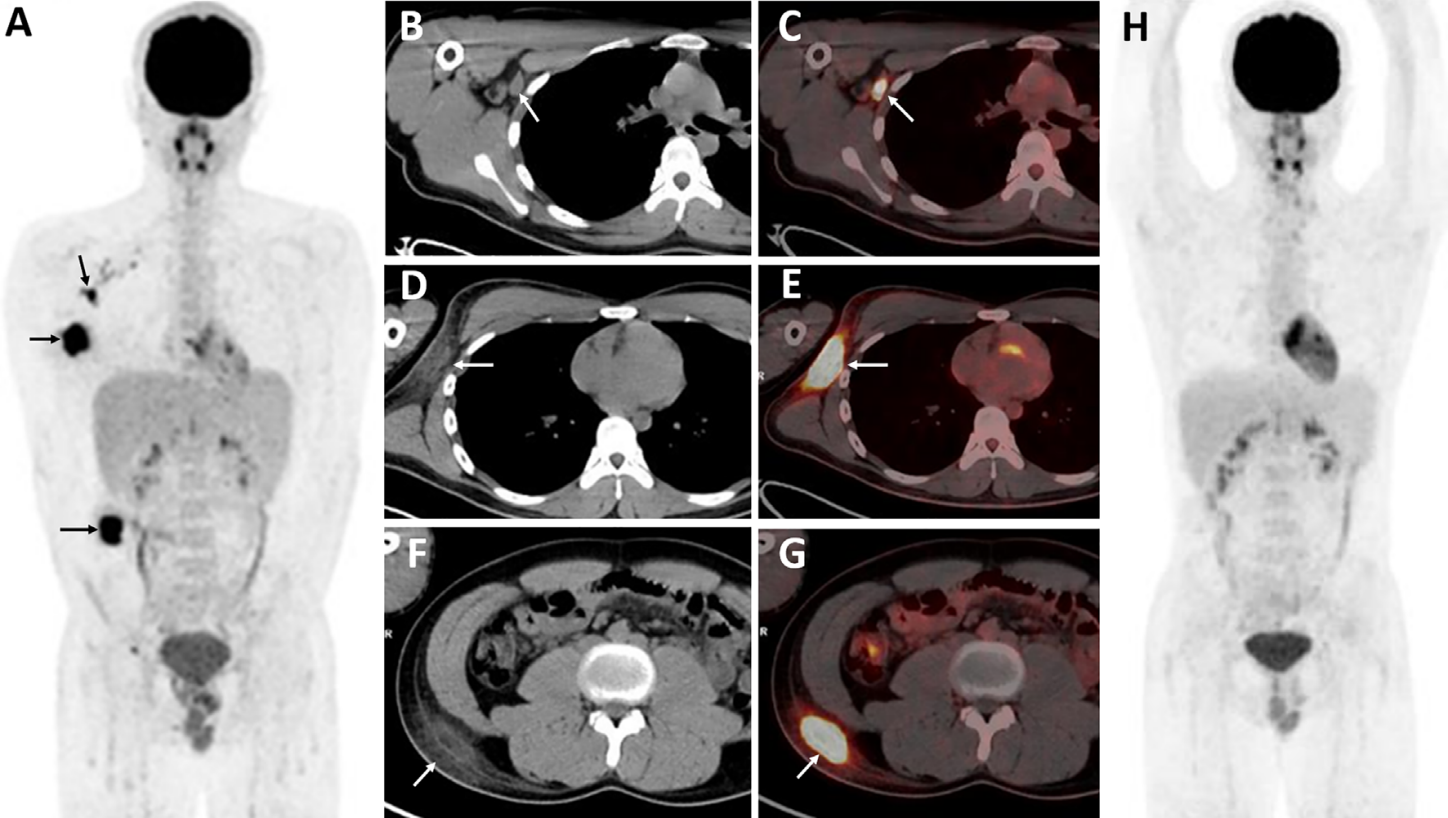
Eighteen-year-old male with subcutaneous panniculitis-like T-cell lymphoma. Three-dimensional maximum intensity projection image (A) shows three intensely ^18^F-FDG-avid lesions in the right side of the body (black arrows). Corresponding axial CT (B, D, F) and fusion PET/CT (C, E, G) images clearly show an enlarged lymph node with increased uptake of ^18^F-FDG in the right axillary and ill-defined lesions in the subcutaneous adipose of the body, representing sites of lymphoma. The patient achieved complete remission after chemotherpay (H).
